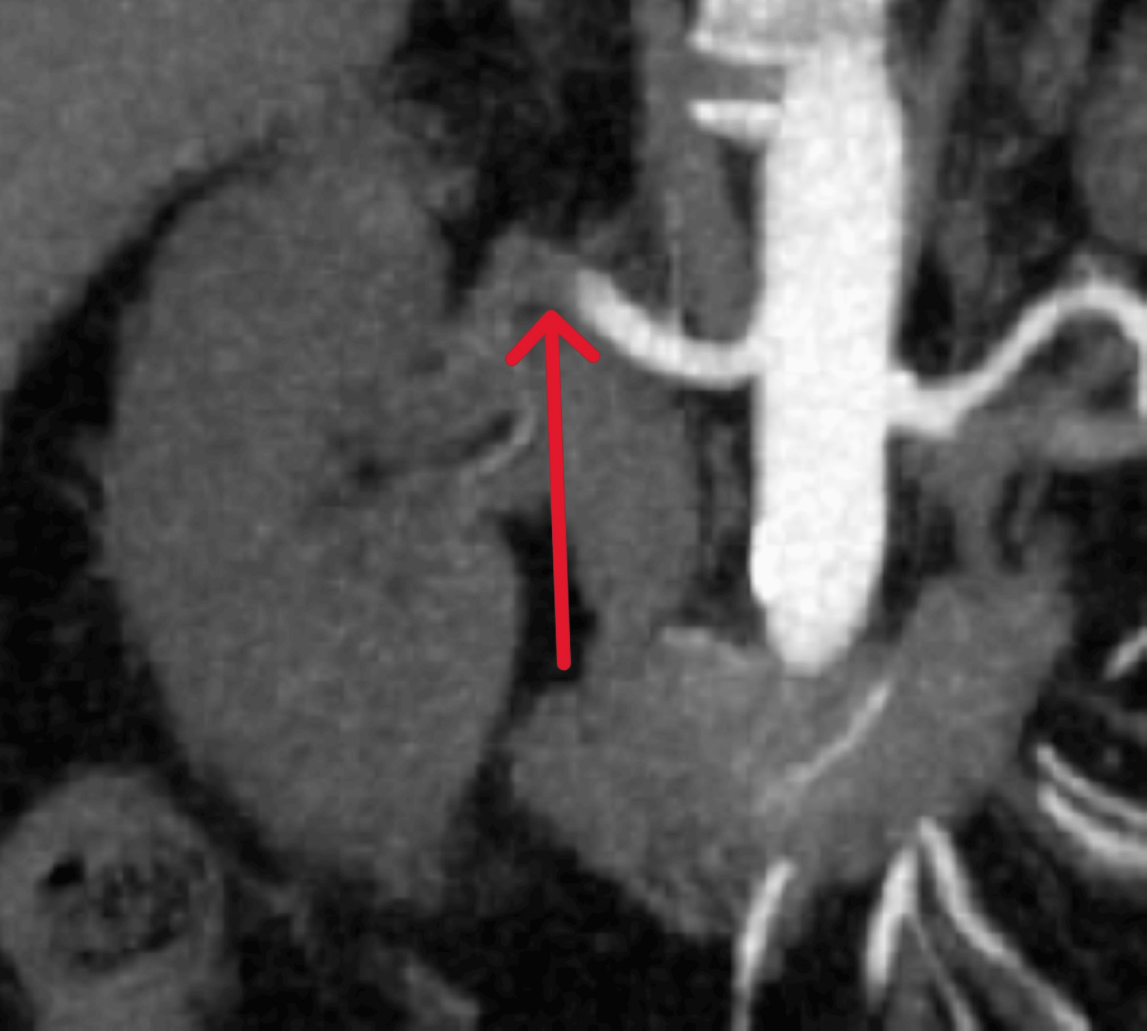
Case 1: Coronal CT image showing a filling defect (red arrow) in the right renal artery, consistent with thrombus
